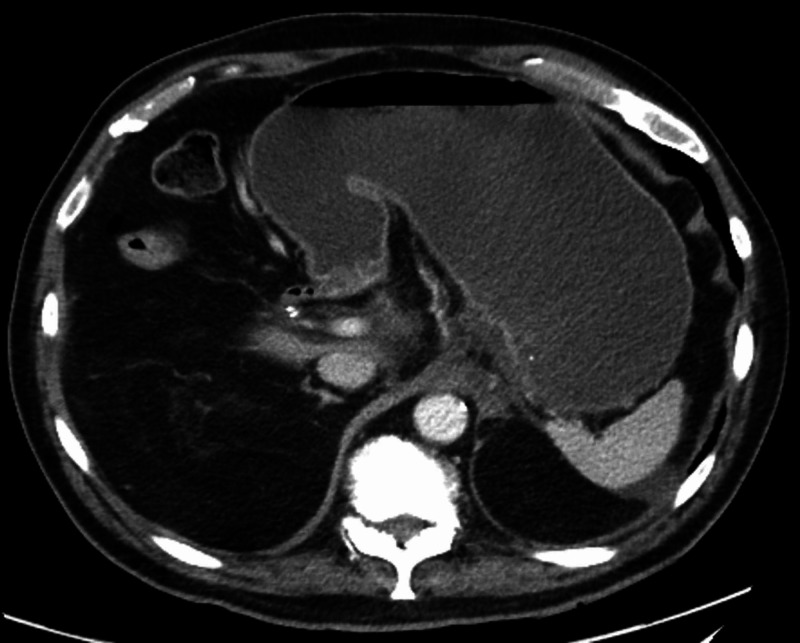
Computed tomography scan of the abdomen showing gastric distension.
